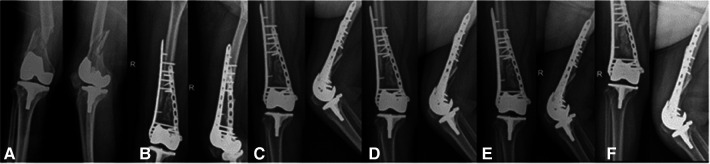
Anteroposterior and lateral radiographs of the right knee: preoperative x-ray image (A), postoperative x-ray images (B), radiograph 7 months after surgery (C), radiograph 9 months after surgery (D), radiograph 21 months after surgery (E), radiograph 34 months after surgery (F).
